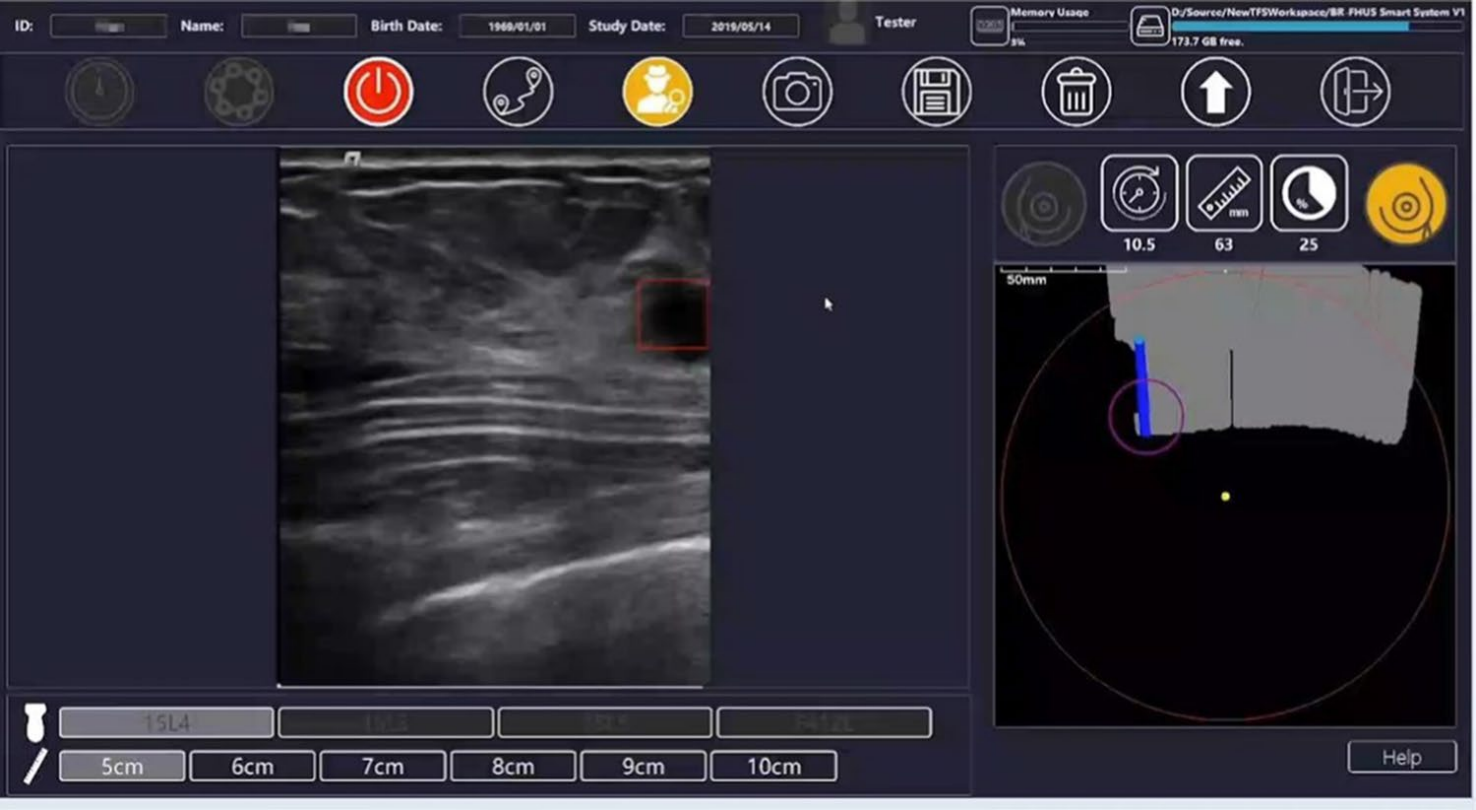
Route map and region of suspected breast cancer displayed by the CADe system, The CADe system; breast free-hand ultrasound (BR-FHUS). The BR-FHUS Smart System is based on AI technology and has several functions. It displays the trajectory of the probe of the ultrasound imaging system as a route map, performs shape analysis during the examination, and immediately visualizes areas suspected of having breast cancer as ROIs on the image screen
